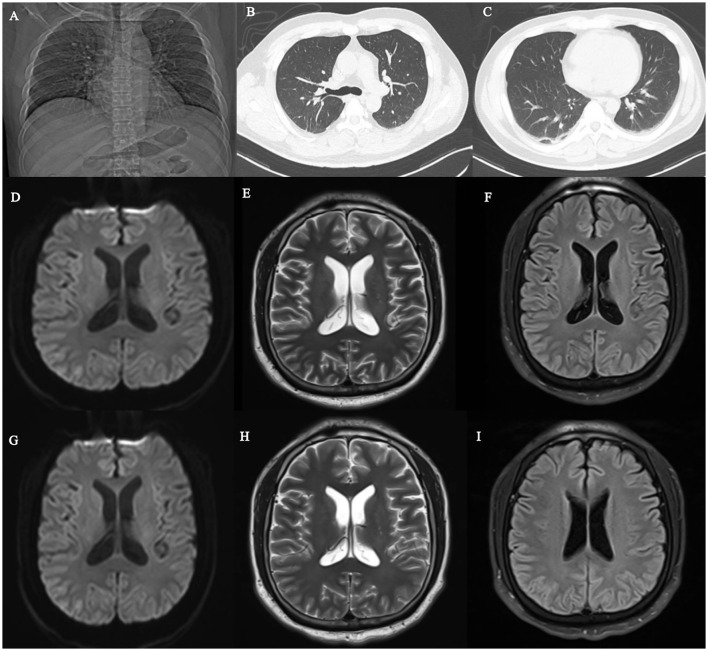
Comprehensive Radiological Findings. (A) Chest X-ray on the first admission showing no acute pulmonary infiltrates. (B, C) Chest CT on the second admission confirming the absence of typical intrapulmonary infiltrates suggestive of Legionella pneumonia. (D-F) Head MRI on the day of admission demonstrating no structural lesions or restricted diffusion on DWI, T2WI, and FLAIR sequences. (G-I) Follow-up head MRI prior to discharge remaining unremarkable, lacking the reversible splenial lesions occasionally associated with Legionnaires' encephalopathy.
